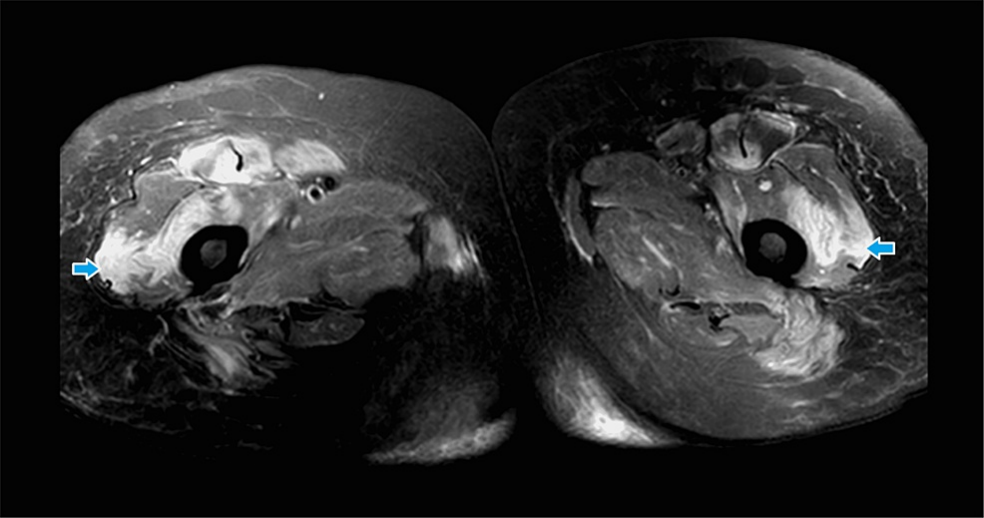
Magnetic resonance imaging (T2-weighted, axial view) of the thighs showing high signals in the muscles.
